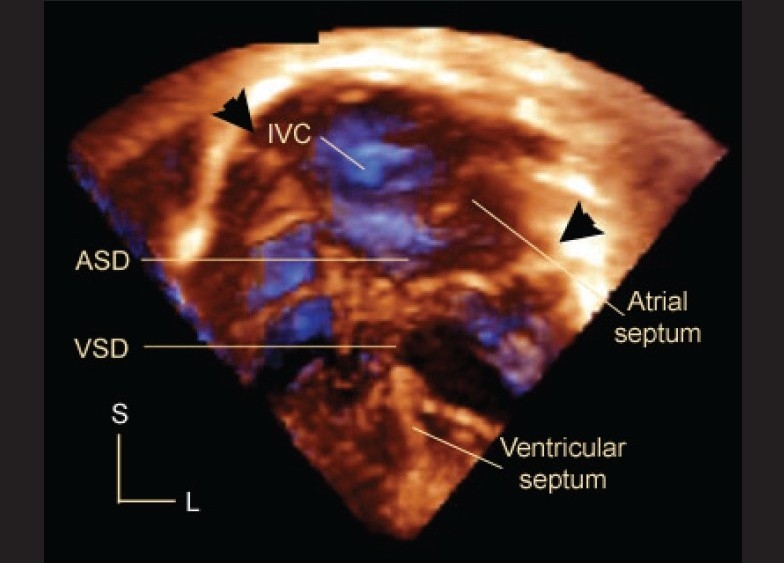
This is an apical four chamber 3D echocardiogram in a patient with atrioventricular septal defect. Arrowheads mark the hinges of the common atrioventricular junction. The bridging leaflets divide the defect into an interatrial (ASD) and interventricular (VSD) component. Note the posterior location of the inferior vena caval orifice (IVC) relative to the septal structures. L, left; S, superior
